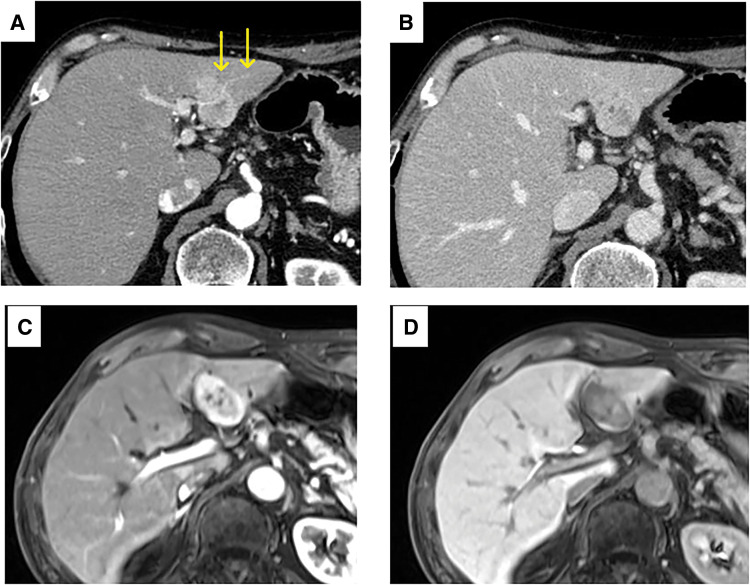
Preoperative imaging findings of the ICC. (A) Contrast-enhanced CT in the arterial phase reveals a tumor with peripheral ring enhancement and a penetrating vessel sign (arrows). (B) Delayed-phase CT demonstrates that the tumor shows iso-enhancement compared with the adjacent parenchyma. (C) Arterial phase of contrast-enhanced MRI also shows peripheral hyperenhancement. (D) Hepatobiliary phase MRI reveals a clear signal defect in the tumor. ICC, intrahepatic cholangiocarcinoma
